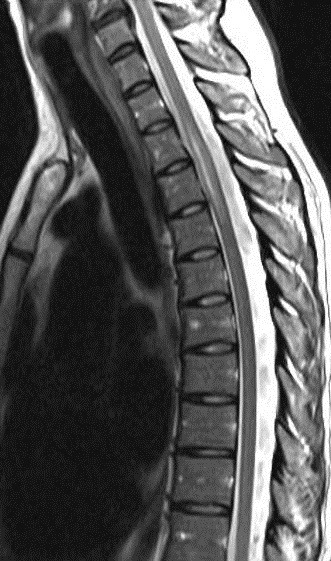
MRI spine showing hyperintensity in the thoracic cord till T12 level (3)
